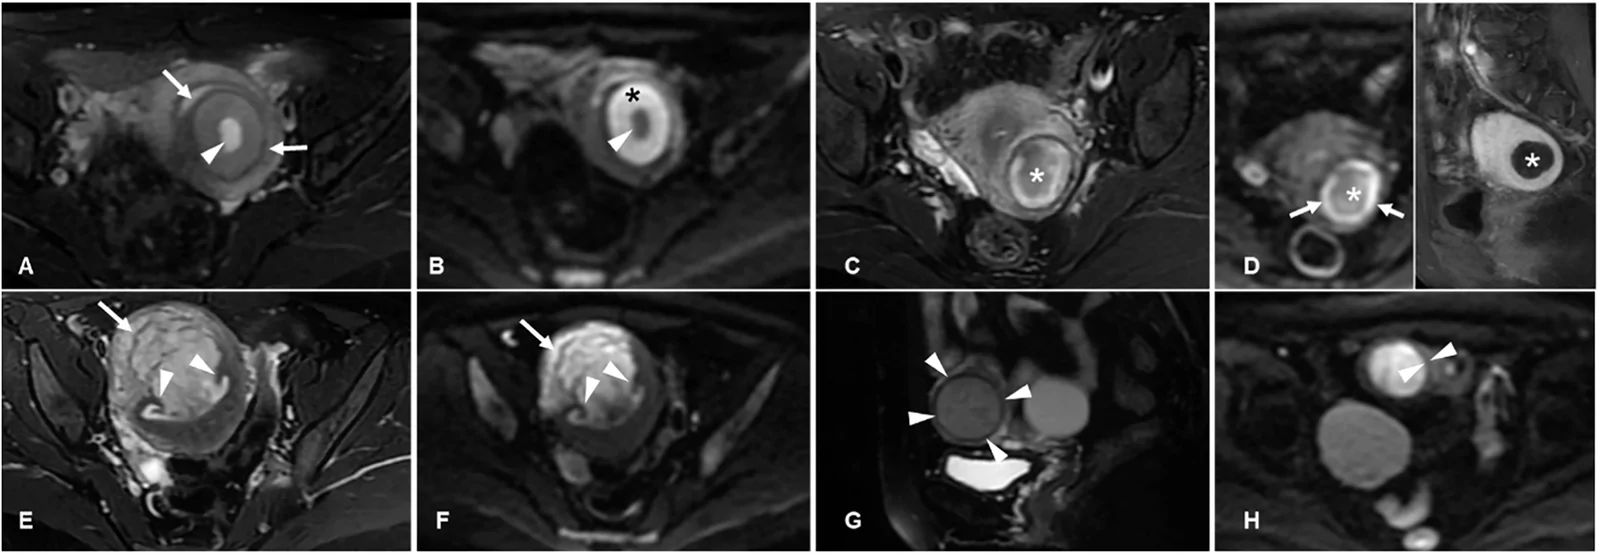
Pelvic magnetic resonance images of LG-ESS in four women: 33 years old (A, B), 46 years old (C, D), 46 years old (E, F), and 51 years old (G, H). Axial T2W (A) and DW (B) images show a well-defined T2WI hypointense rim (arrow) around the lesion, with a central oval cystic area (arrowheads) surrounded by DWI-hyperintense tumor tissue (asterisk). Axial T2W (C), axial DW, and sagittal contrast-enhanced T1-WI (D) reveal a well-defined posterior wall mass. A central round T2WI hypointense/DWI hypointense nonenhancing area (asterisk) indicates necrosis, encircled by a DWI-hyperintense tumor (arrows). Axial T2W (E) and DW (F) images show a mass in the anterior uterine wall eroding through the myometrium (arrowheads), with the disrupted myometrial bundles forming short, band-like T2WI/DWI hypointense structures angling toward the endometrial cavity. Multiple T2WI/DWI hypointense bands are also visible within the tumor (arrow). Sagittal T2WI (G) and axial DWI (H) depict a well-circumscribed round mass in the right uterine wall. A peripheral T2WI/DWI-hypointense rim (arrowheads) surrounds the lesion
